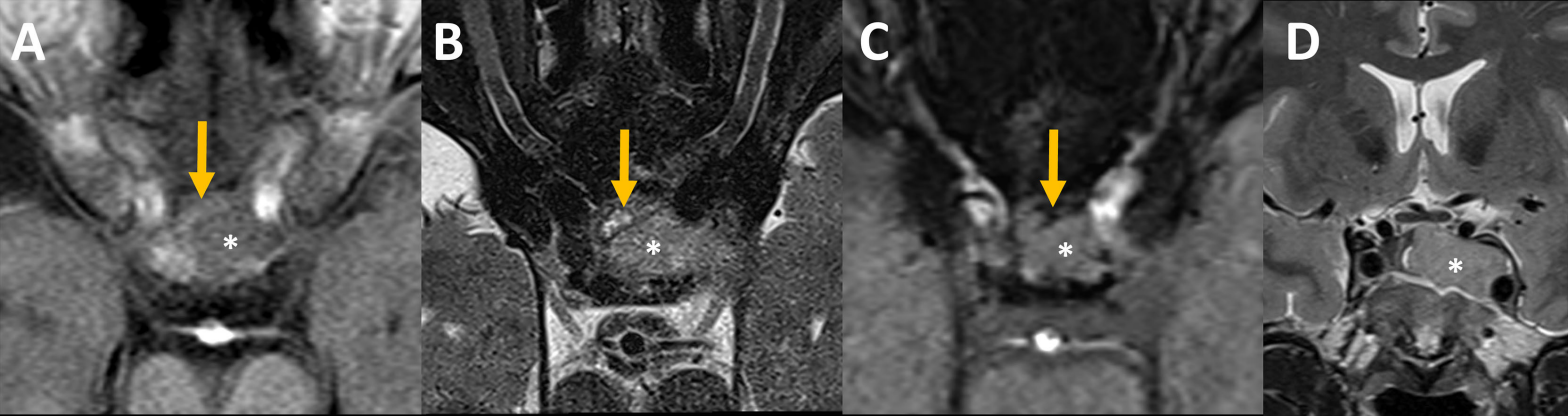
Pre-operative MRI Axial MR imaging focused on the sella at admission (A–C): The intra-sellar lesion (star) was heterogeneous on its anterior aspect. Some bloody components (orange arrows) that appeared slightly hyperintense on unenhanced T1 (A), hyperintense with an hypointense rim on T2 spin echo (B) and hypointense on susceptibility weighted images, SWI, (C) were intercalated between the bone and the rest of the lesion. (D) Coronal MR imaging showed pituitary adenoma with invasion of the left cavernous sinus, grade 3b according to Knosp classification (17).
